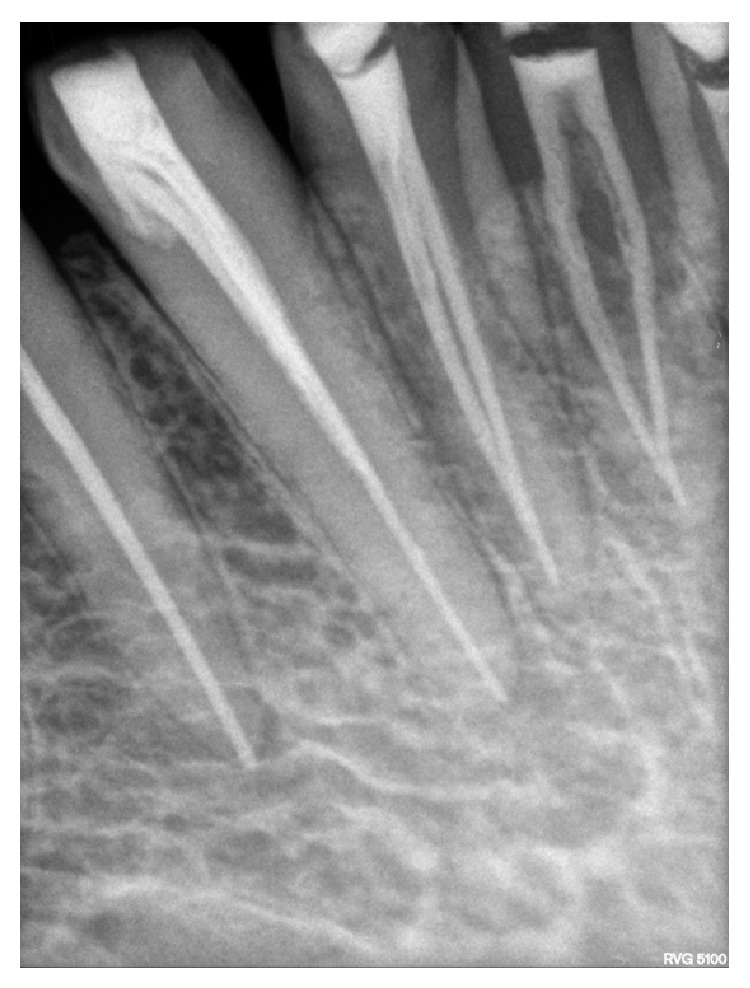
Radiograph showing obturation in two separate canals in 31 and 32.
